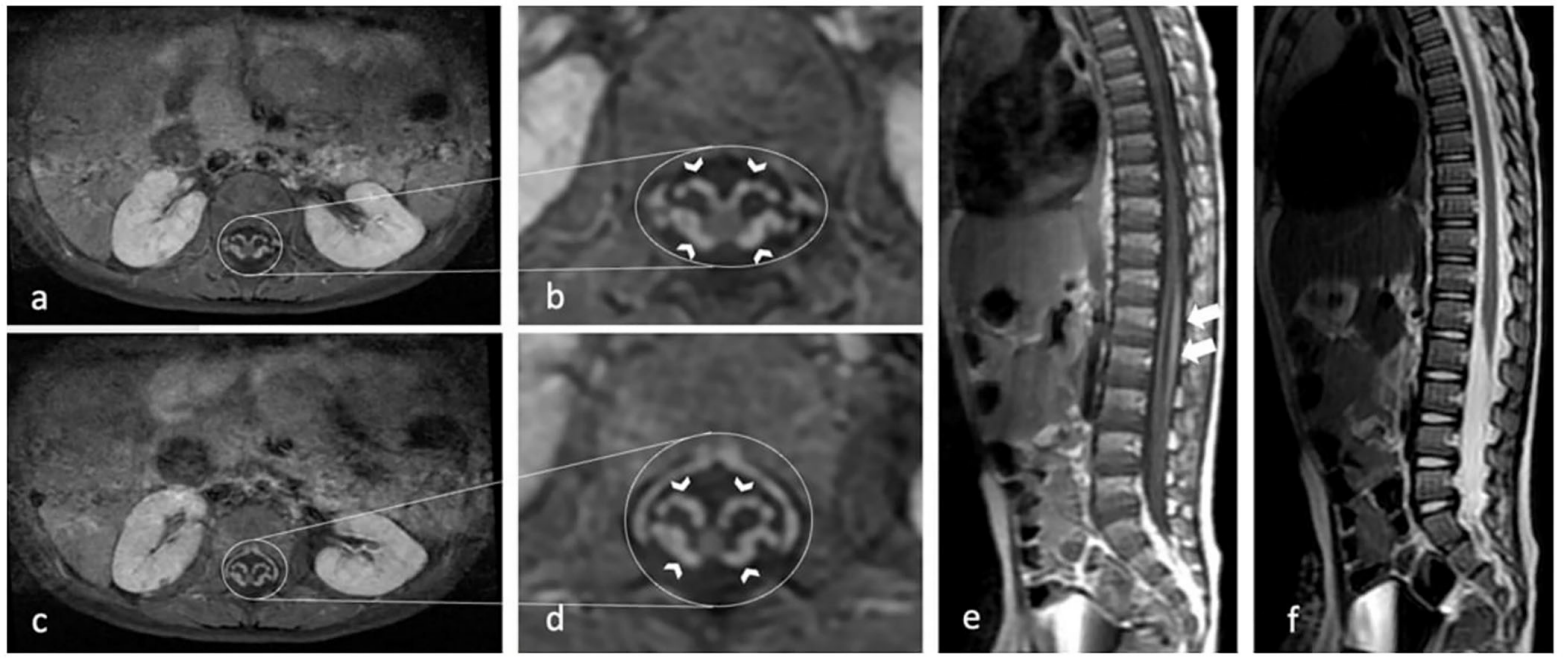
Contrast-enhanced axial T1-weighted MR image shows marked enhancement of the anterior and posterior nerve roots (arrow heads) in the conus medullaris and cauda equina (a–d). Sagittal T1-weighted MR image (e) show mild thickening and moderate contrast enhancement of nerve root in the conus medullaris and cauda equina (white arrows). Sagittal T2-weighted MR image (f) show mild thickening of cauda equina.
